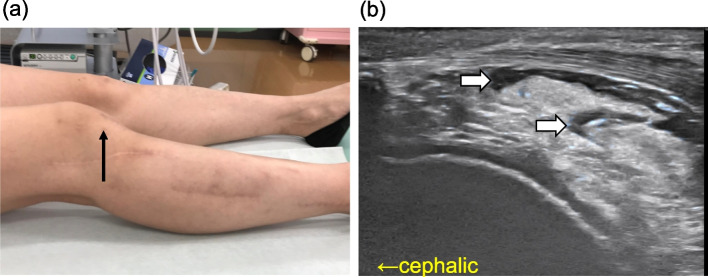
Injection into the infrapatellar fat pad. a Injection site (black arrow). The needle entry point is a 3-cm lateral from the center of the patellar tendon. b Ultrasound image after injection of 0.2% lidocaine 3 ml into each of the infrapatellar fat pad and the space between the patellar tendon and infrapatellar fat pad. Echo-free spaces (white arrow) are formed by local anesthetics
